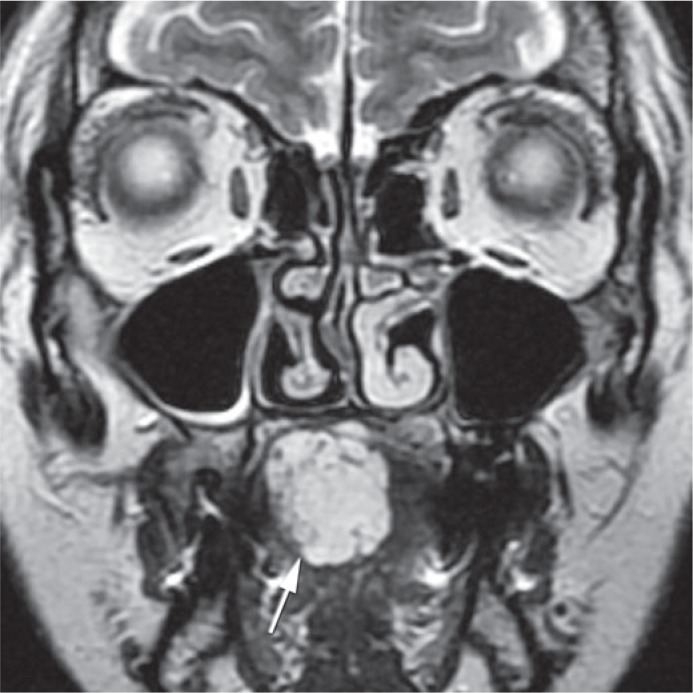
T2 weighted MR image of the same lesion (white arrow) in coronal plane before treatment shows that the majority of lesion is located on the left side of the tongue.
